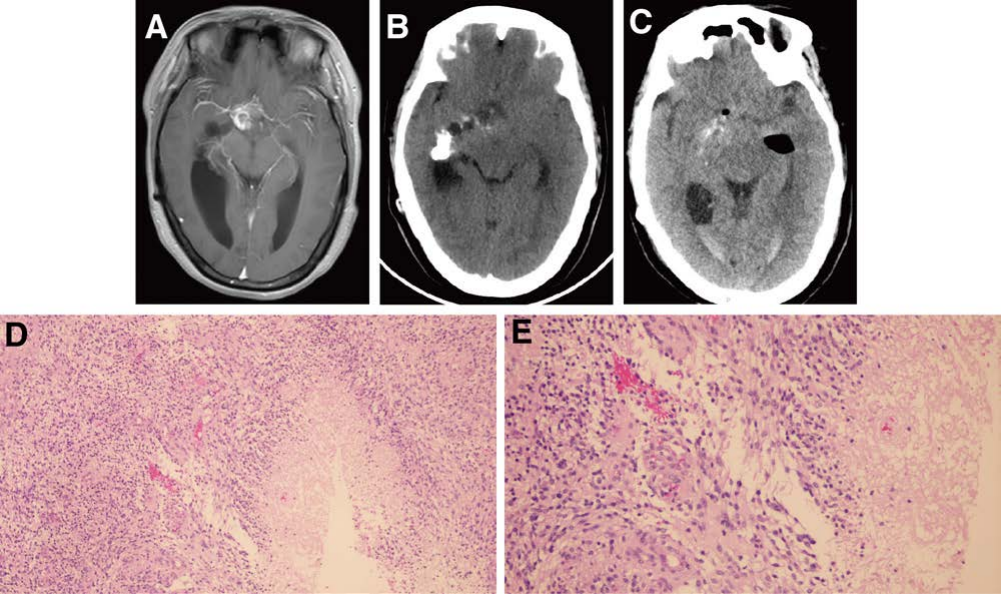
Clinical data of patient number 3. (A) an irregular, patchy isointense T1 and slightly hyperintense T2 signal was observed in the right lateral ventricle and septum pellucidum-foramen of Monro region. The signal was heterogeneous; FLAIR showed uneven hyperintensity, DWI showed high signal intensity, and ADC showed low signal intensity. Contrast-enhanced imaging demonstrated markedly heterogeneous enhancement of the lesion. (B) Preoperative CT revealed irregular, mass-like mixed hyperdense and hypodense lesions in the midline region between bilateral lateral ventricles, within the right lateral ventricle, basal ganglia, and right temporal insular lobe. The lesion margins were ill-defined, with intralesional clump-like calcification and cystic changes. (C) Postoperative CT showed irregular, mass-like mixed hyperdense and hypodense lesions in the midline region between bilateral lateral ventricles, within the right lateral ventricle, basal ganglia, and right temporal insular lobe. The lesion margins remained ill-defined, with clump-like calcification and cystic changes. (D, E) Tumor cells with oval nuclei and high cellularity showed moderate atypia. Pseudopalisading necrosis and microvascular proliferation were observed (left, ×100). Tumor cells demonstrated moderate atypia with visible mitotic figures, along with pseudopalisading necrosis and glomeruloid microvascular proliferation (right, ×200). ADC = apparent diffusion coefficient, CT = computerized tomography, DWI = diffusion weighted imaging, FLAIR = fluid attenuated inversion recovery.
